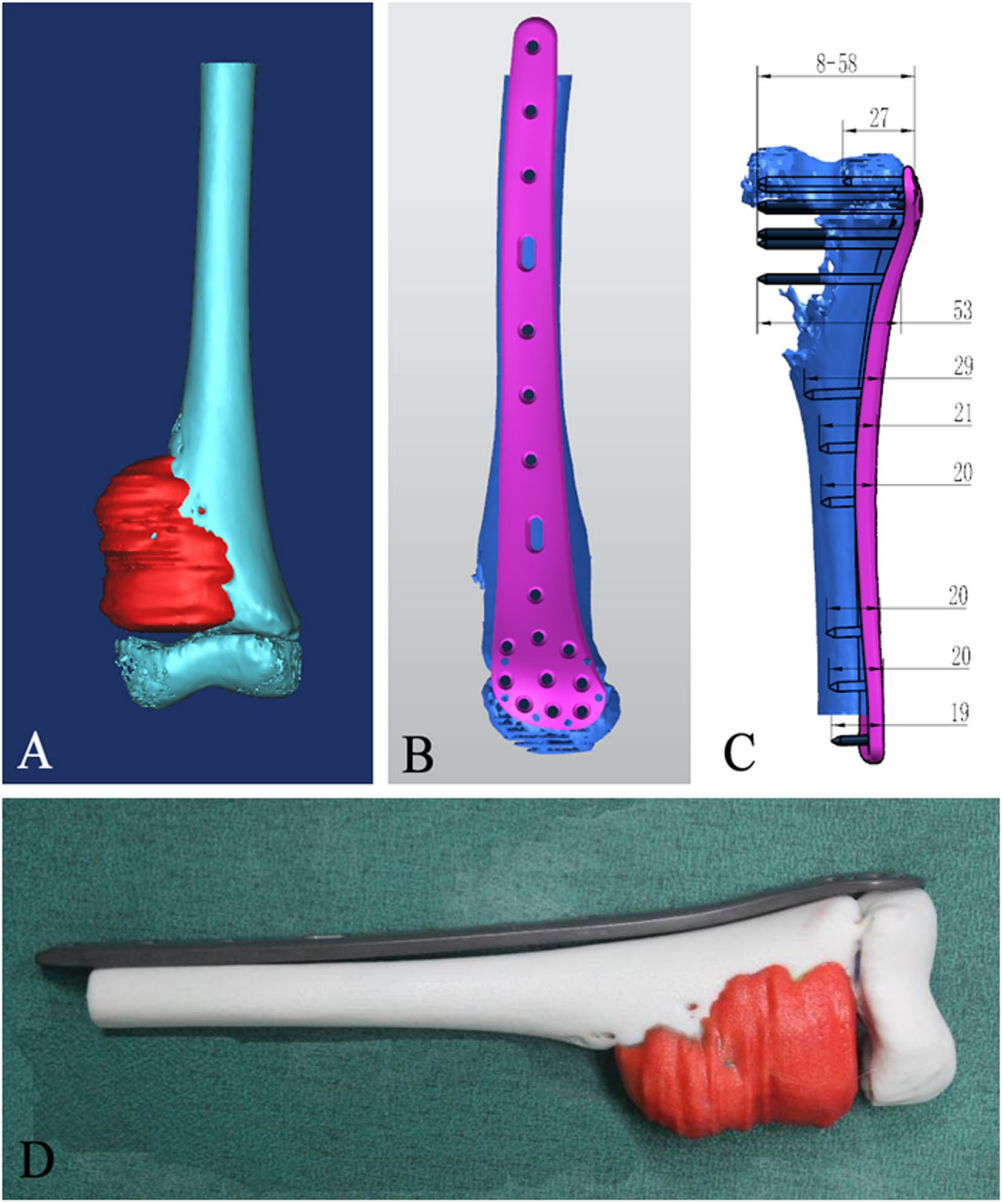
The modeling of the tumorous bone and personalized titanium plate as well as the matching between the tumorous bone and the titanium plate. (A) The 3D model of the tumorous bone was constructed with Mimics software according to the merged CT-MR data. (B) The 3D model of the tumorous bone is matched with a personalized titanium plate model (pink) using UG software. (C) The resultant 3D model is filled with screws in order to show the required number as well as the location and lengths of the screws that will be used during the operation. (D) The 3D printed tumorous bone model is matched with a fabricated personalized titanium plate.
